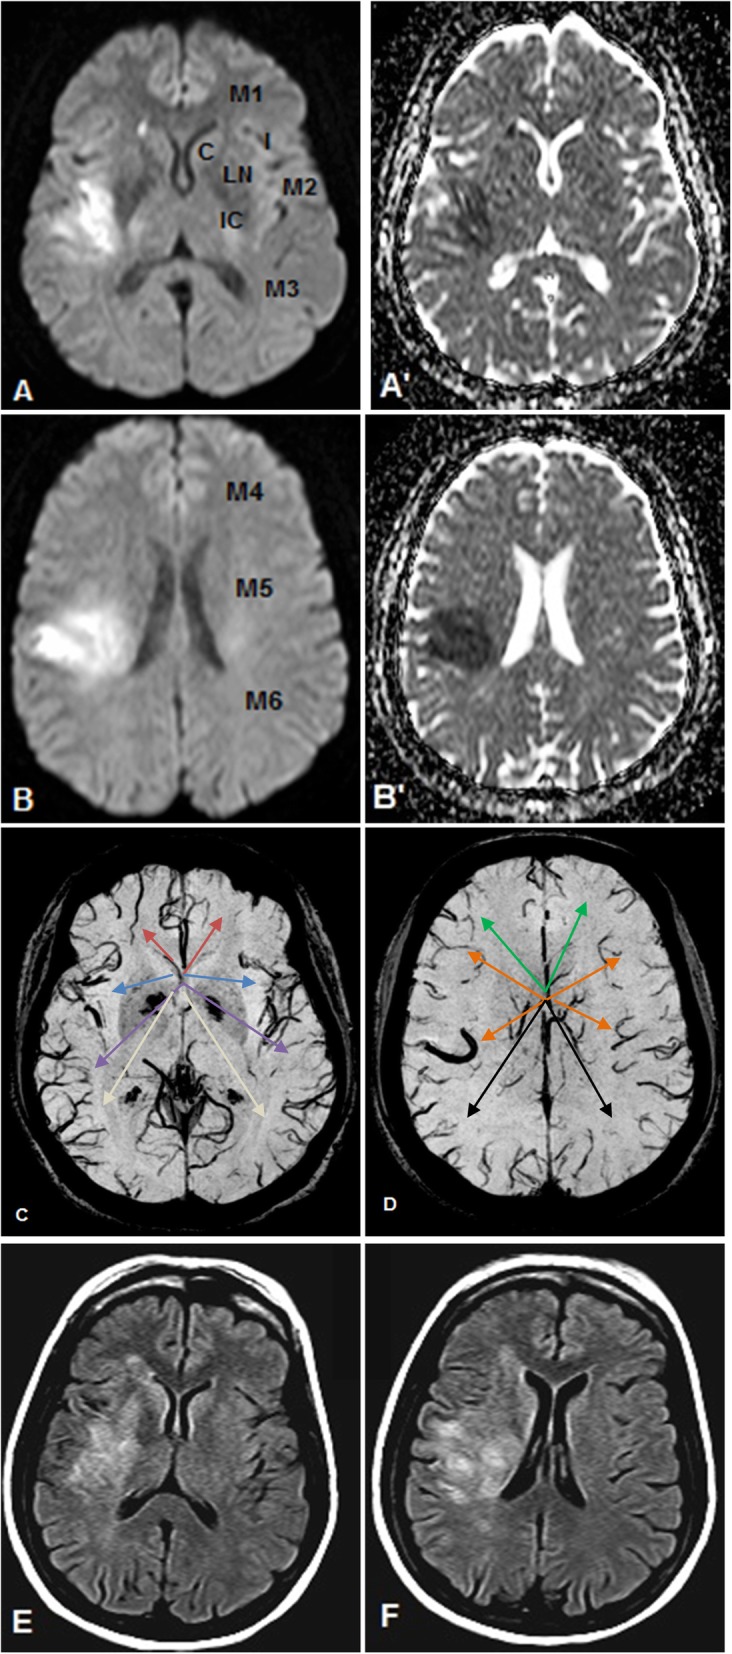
a–f 60-year-old female with right MCA territory infarct. The 10 MCA zones designated by the ASPECTS scoring system are *C* caudate, *L* lentiform nucleus, *IC* internal capsule, *I* insular ribbon, *M1–6* cortical regions (M1–3 at the level of basal ganglia, M4–6 at the level cranial to the basal ganglia). Initial DW images and their corresponding ADC maps at the basal ganglia level (a, a') and the supraganglionic level (b, b') reveal the presence of acute infarction in I, M1, M2, and M5 regions with a resultant ASPECTS score of 6. SW images at the basal ganglia level (c) and the supraganglionic level (d) reveal the presence of APVs, in terms of either density and/or caliber and/or hypointensity, in I (blue arrow) and in M1–6 regions (red, purple, gray, green, orange, and black arrows respectively) with a resultant ASPECTS score of 3 (similar colored arrows point to the corresponding areas in the contralateral normal hemisphere for comparison). DWI/SWI mismatch score is 3. Follow up FLAIR images, obtained 10 days later, at the basal ganglia level (e) and the supraganglionic level (f) reveal in addition to the originally infarcted zones, infarction growth in L, IC, and M4, giving an overall ASPECTS score of 3 and an IGS of 3. The faint hyperintensities noted at M3 and M6 zones were considered to be ischemic foci and not newly infarcted areas as they were evident in the FLAIR images of the initial MRI with no corresponding diffusion restriction
